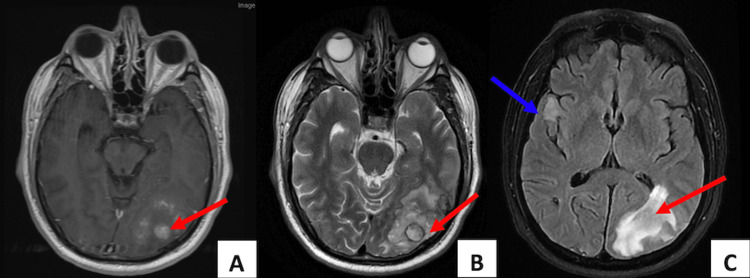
T1 (A), T2 (B), and flair (C) MRIs of the brain reveal a circumscribed mass (red arrows) in the left occipital lobe that is contrast-enhanced and with surrounding edema. Multiple ischemic or hemorrhagic lesions were noted in the cerebral hemispheres and cerebellum (blue arrow). MRI: magnetic resonance imaging
